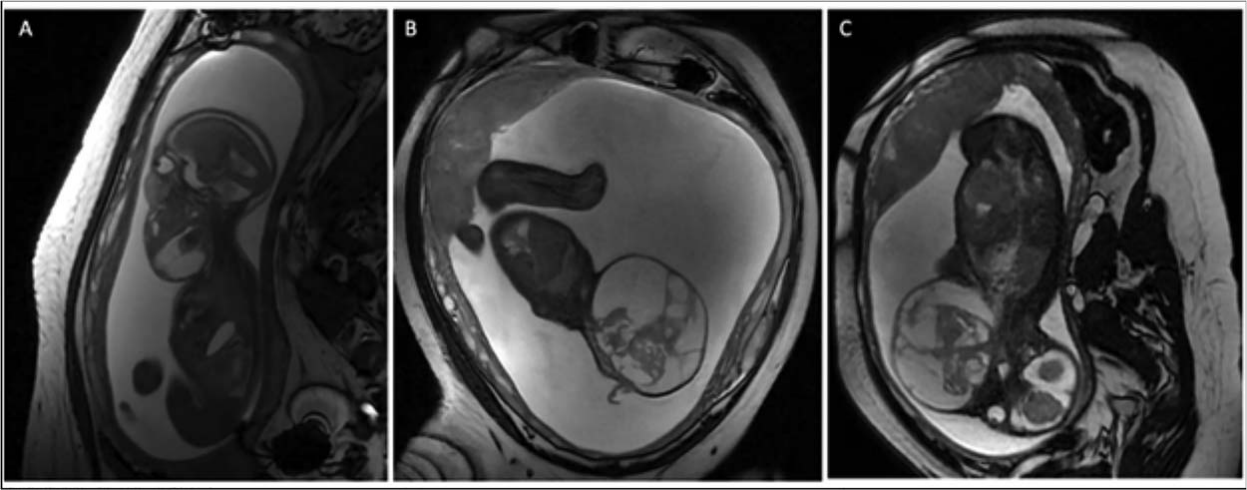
(A) Sagittal T2 weighted fetal MRI at 21 weeks gestation with fetal mass volume of 33 ml (3.7 × 4.7 × 3.6 cm). (B) Sagittal T2 weighted fetal MRI at 29 weeks gestation with fetal mass volume of 411 ml (10.3 × 9.3 × 8.2 cm). (C) Coronal T2 weighted fetal MRI at 29 weeks demonstrating fetal neck hyperextension due to mass effect.
